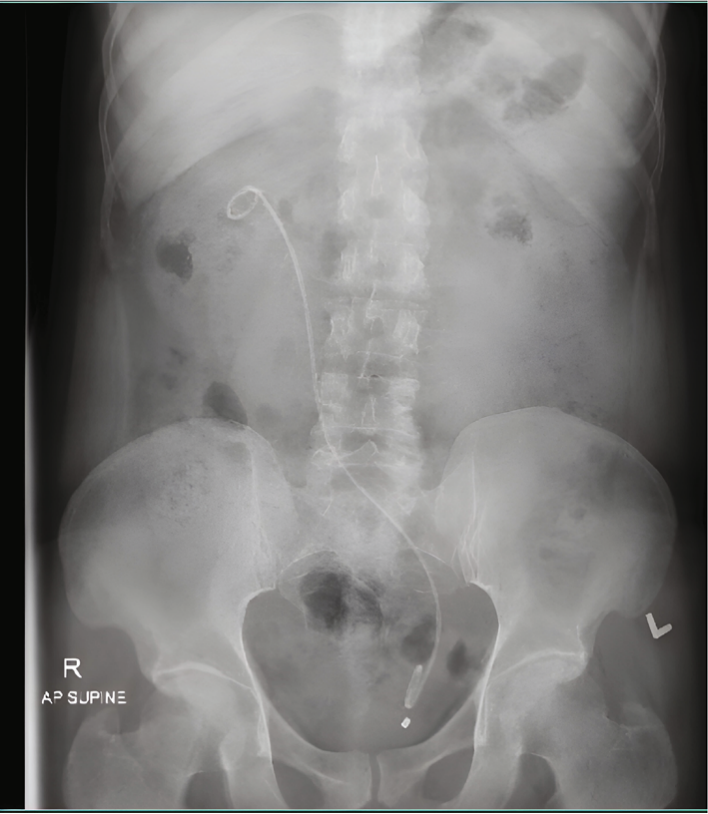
Postoperative x-ray of the abdomen showed a left double-J stent in place crossing to the right side.
